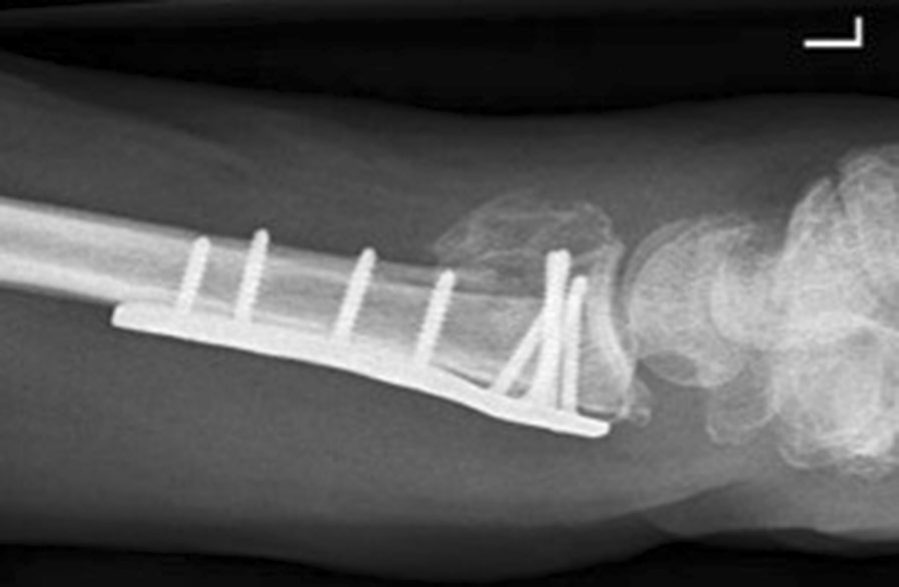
Bent volar locking plate. Lateral wrist radiograph of an operatively treated distal radius fracture patient after a new fall causing a re-fracture and bending of the volar locking plate. This was a new trauma and therefore not accounted for as a complication
